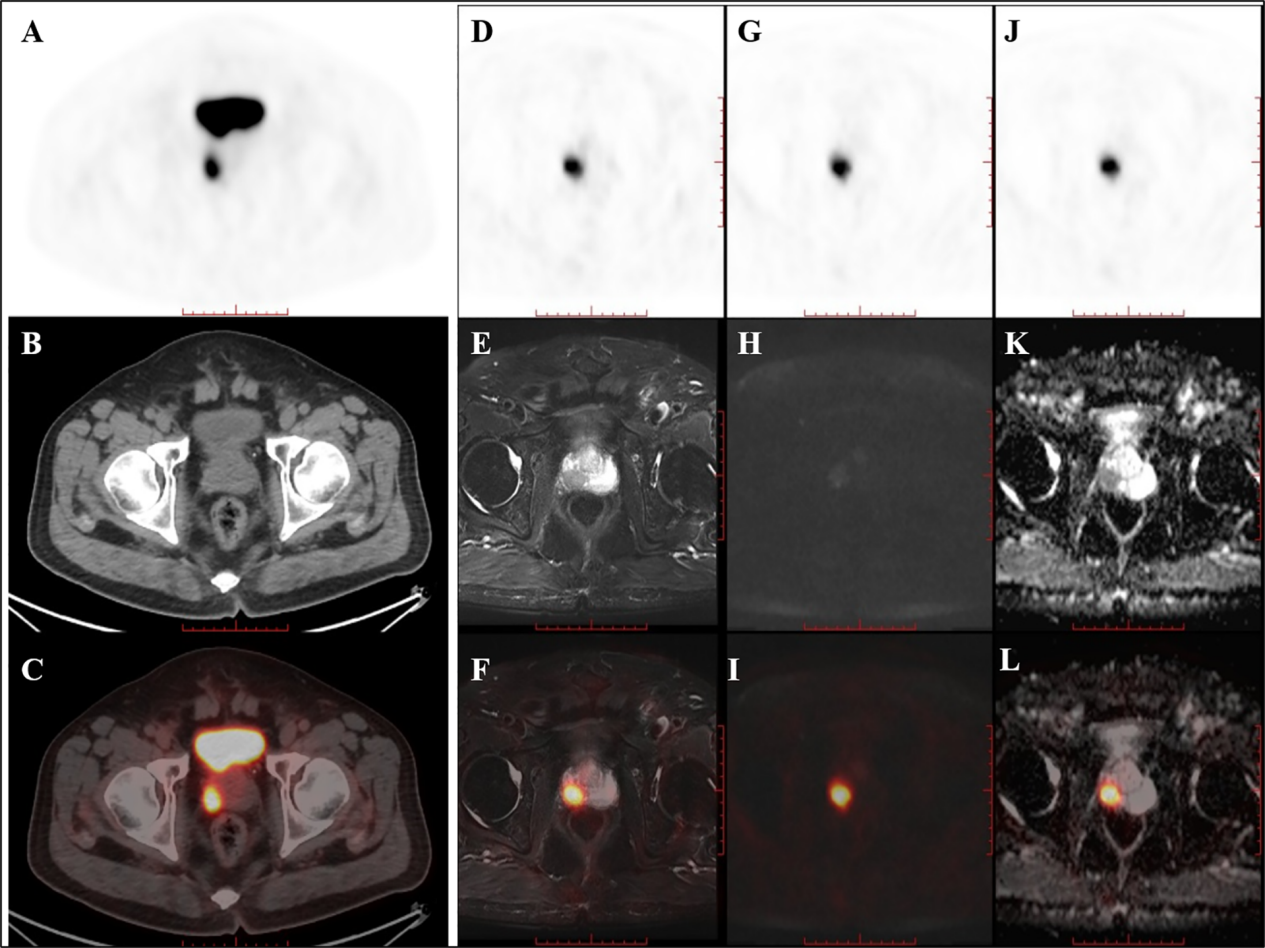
PET/CT found one PET-positive lesion in the prostate gland (A: PET, B: CT, C: fused PET/CT). PET/MRI showed short T2 signal (D: PET, E: T2WI, F: fused PET/T2WI), high DWI signal (G: PET, H: DWI, I: fused PET/DWI), and a decreased ADC value at the site of the PET-positive lesion (J: PET, K: ADC map, L: fused PET/ADC map). The subsequent pathology confirmed prostate cancer.
